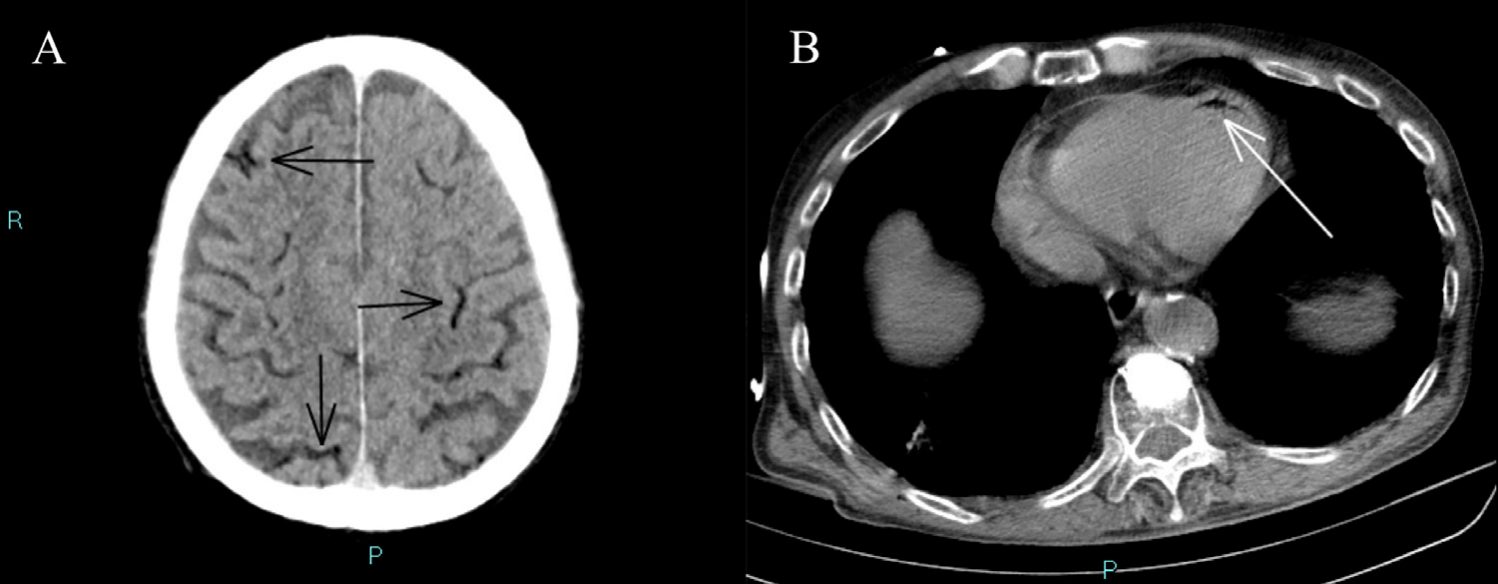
CT showing the presence of air microbubbles along the subarachnoid spaces of the bihemispheric convexity (black arrows in A) and in endocardial profile of the left ventricle (white arrow in B).
